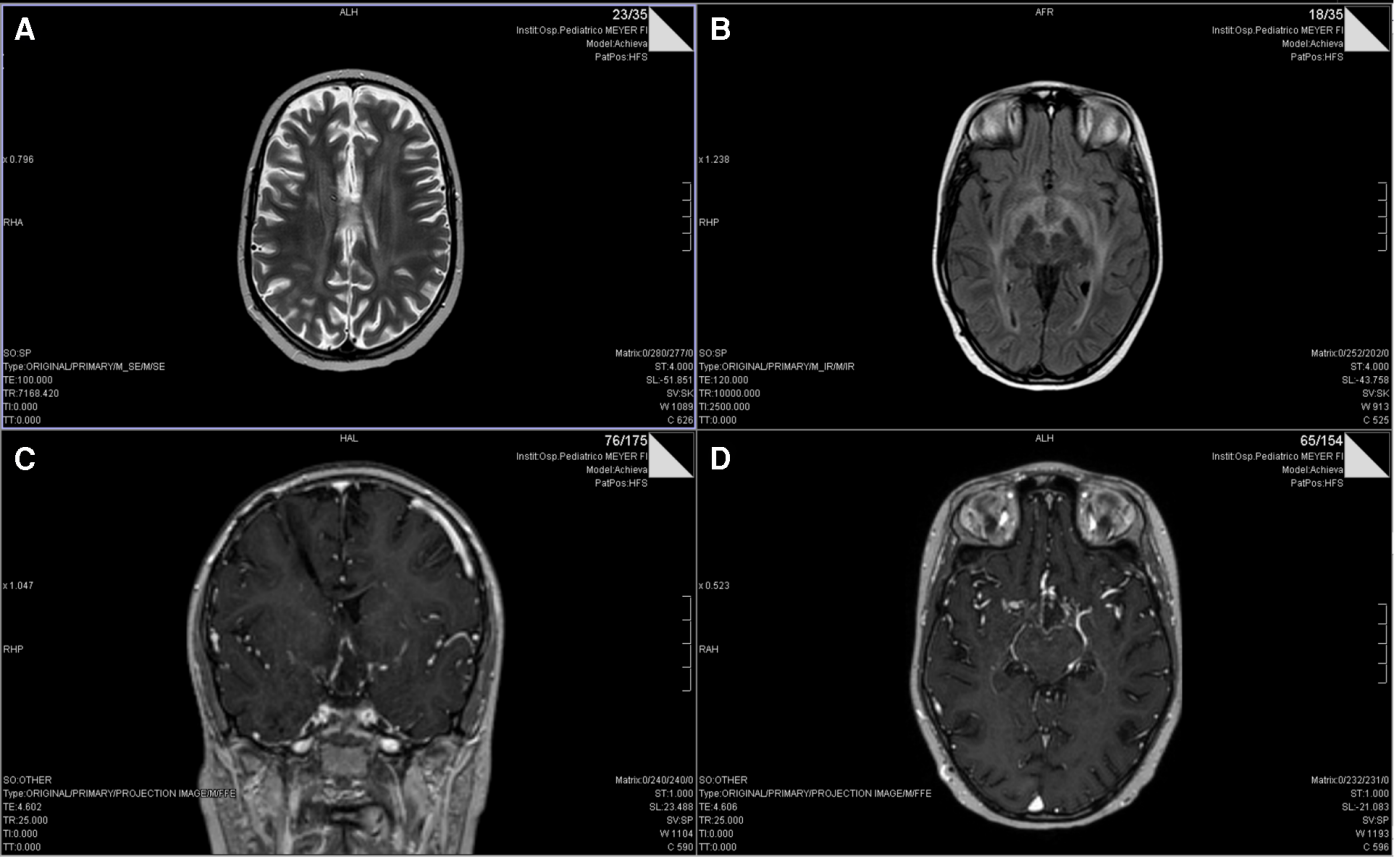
T2 (A) and FLAIR (B) axial MRI scans showing multifocal leukoencephalopathy after two months of maintenance treatment. Gadolinium-enhanced T1-weighted MRI (C, coronal section; D, axial section) shows a ring enhancement of the hypothalamic lesion compressing the chiasm and the optic tracts.
